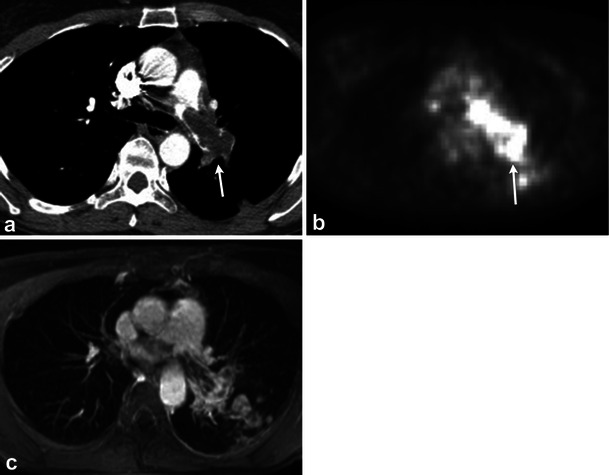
Contrast-enhanced axial CT image (a) demonstrates a large filling defect in the left pulmonary artery (*arrow*). The lesion remained stable after a course of anticoagulation, which raised the suspicion for a malignancy. Subsequently obtained FDG-PET (b) demonstrated the central part of this filling defect to be hypermetabolic, consistent with a primary pulmonary artery sarcoma. Gadolinium-enhanced cardiac MR (c) performed 60 s post contrast for preoperative evaluation demonstrates a lesion in the left pulmonary artery with an non-enhancing central portion, consistent with a bland thrombus, and an enhancing component in the pulmonary arteries and left lower lobe, suggestive of a tumour
